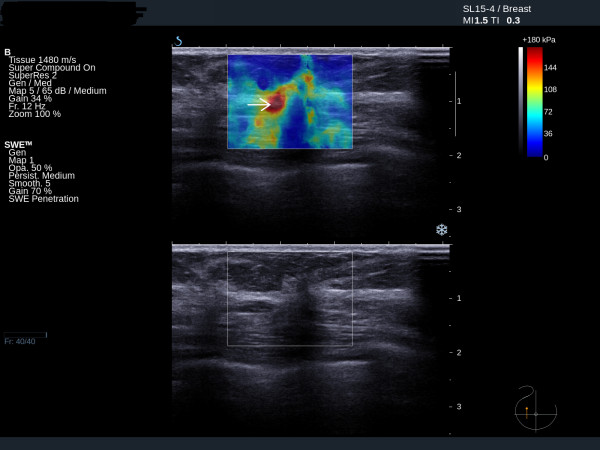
Ultrasound and elastography images of an invasive ductal cancer. Ultrasound and elastography images of an invasive ductal cancer showing typical peri-tumoural stiffness (arrow).
